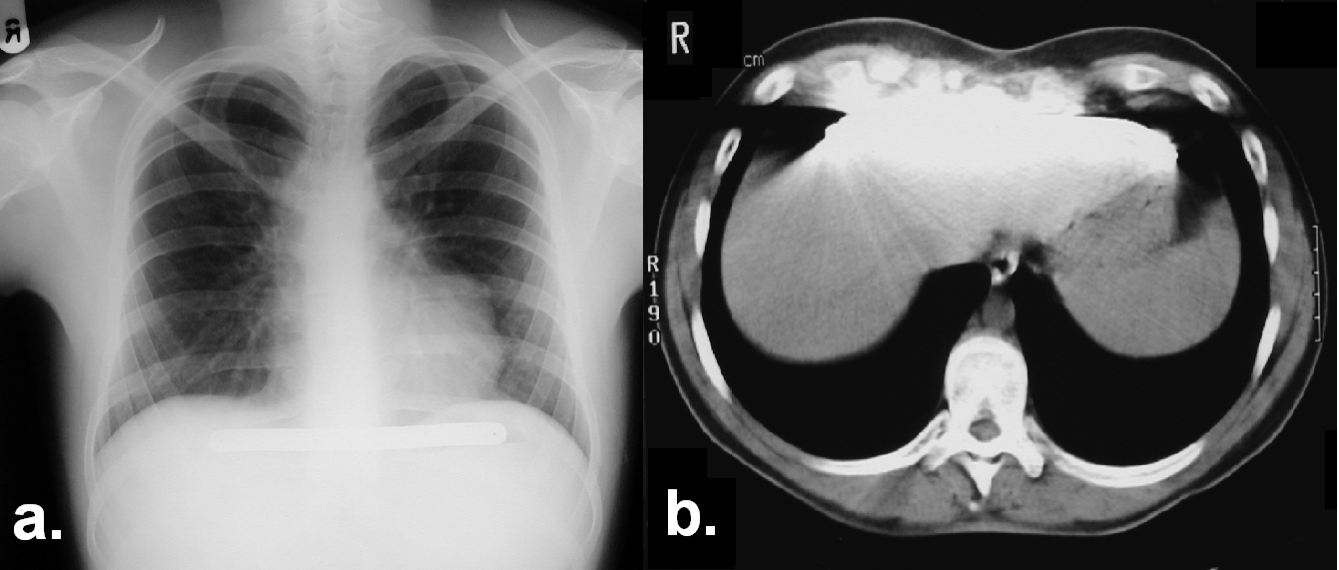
1a Preoperative chest x-ray revealing the pectus bar at the level of the diaphragm. 1b. Subsequent CT imaging of the bar in relation to the liver.
